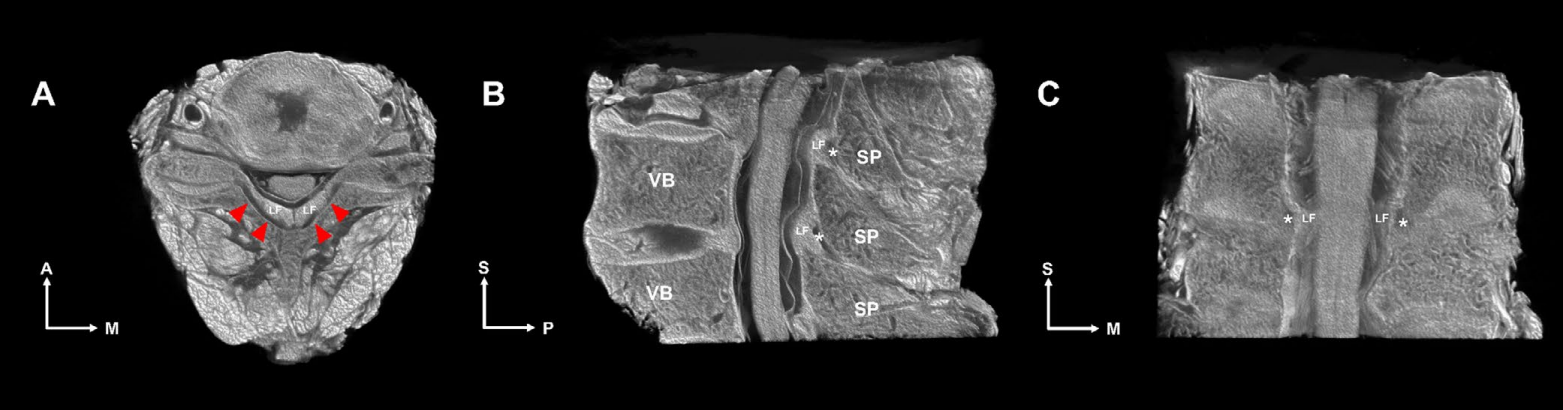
Micro‐CT images of the cervical vertebrae (C5‐7 levels) show the LF and the RSO. In the axial view (A), red arrowheads indicate the RSO, located between the LF and the vertebral lamina. The RSO connects to the epidural space anterior to the LF via a median gap. In the mid‐sagittal view (B), the RSO (indicated by an asterisk) extends posteriorly and inferiorly between adjacent spinous processes. In the coronal view (C), the RSO (indicated by an asterisk) extends to the facet joint. A, anterior; M, medial; P, posterior; S, superior; SP, spinous process; VB, vertebral body.
